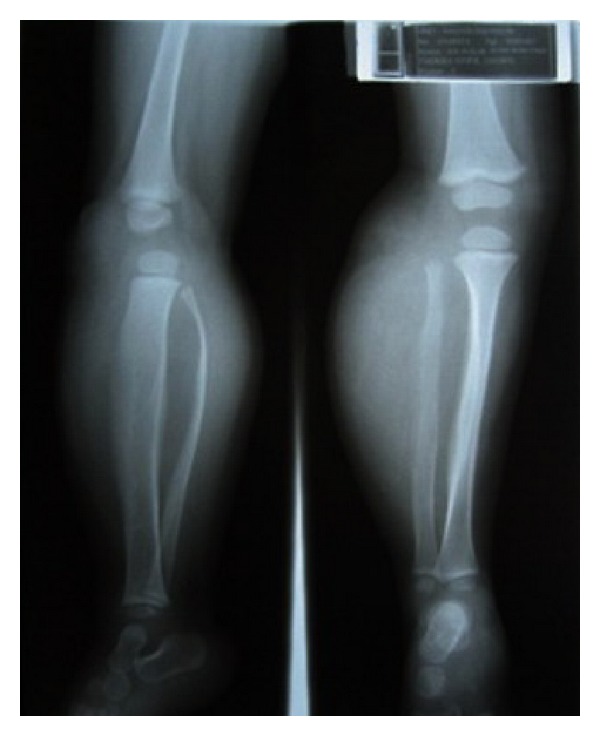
Plain radiograph of Case  1 showed a soft tissue mass. Both tibia and fibula were deformed, which gave an impression of a benign slow-growing lession.
